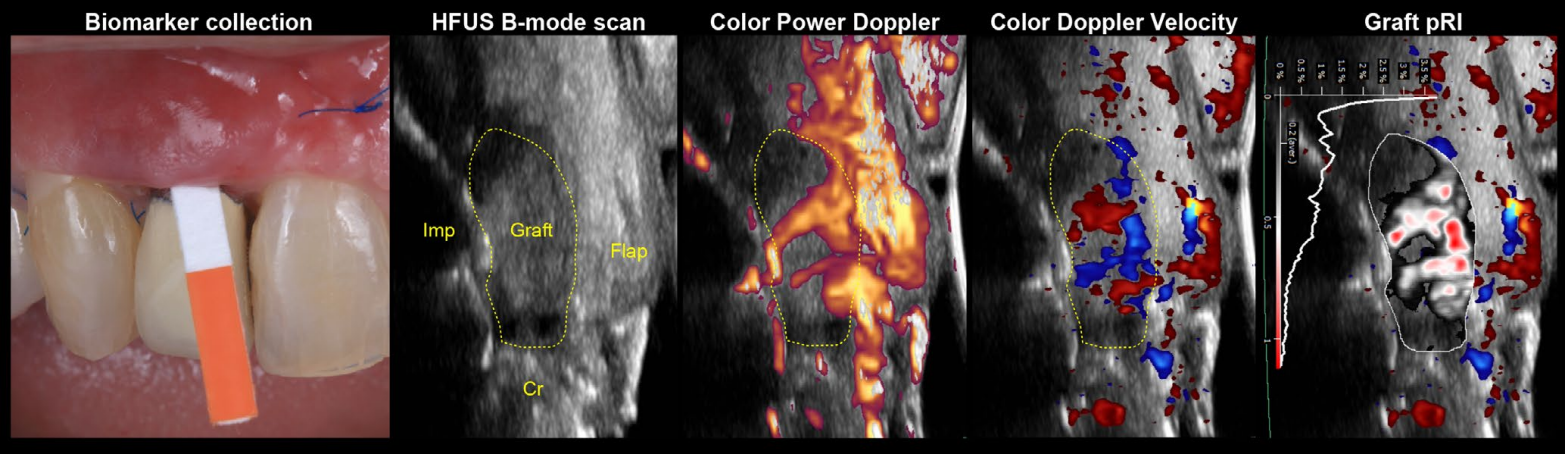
Collection of the peri‐implant crevicular fluid at 1 week and related high frequency ultrasonographic (HFUS) scans recorded at the midfacial aspect. It is possible to appreciate the implant‐supported crown (“Cr”), the implant fixture (“Imp”), the graft, and the flap. Doppler ultrasonographic blood flow evaluation included Color Power Doppler, Color Doppler Velocity, and dynamic tissue perfusion assessment. In particular, the right panel displays the dynamic tissue perfusion evaluation in terms of perfusion relief intensity (pRI) of the connective tissue graft after 1 week. pRI refers to the number of pixel within the region of interest (graft) that relate to the intensity of the respective value. Areas with zero intensity/perfusion within the ROI are shown in black, whereas areas with half of the maximum intensity/perfusion and maximum intensity/perfusion are visualized in white and red, respectively.
